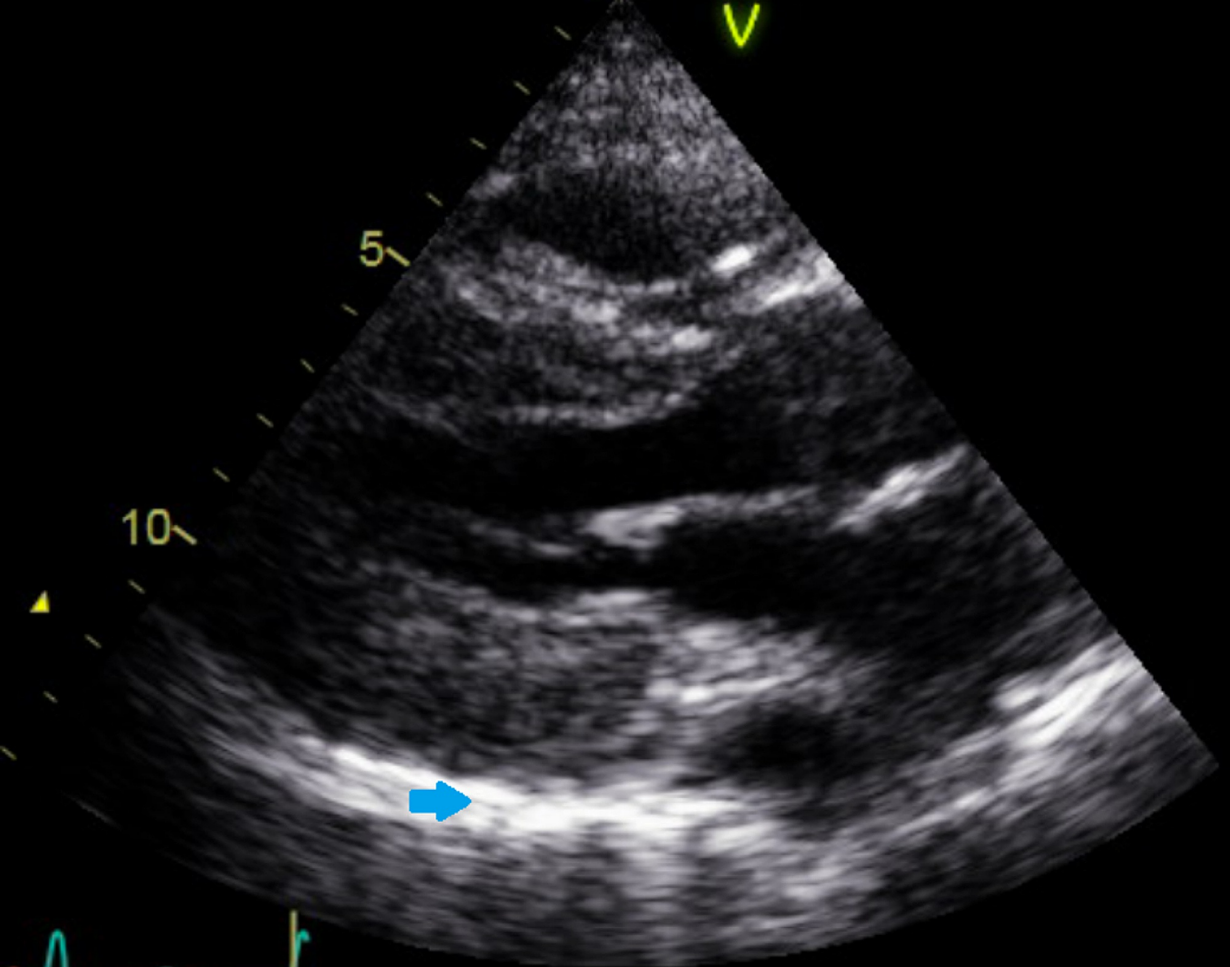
Echocardiogram after pericardiocentesis. Blue arrow: indicating pericardium, now with resolution of the previously seen effusion.
